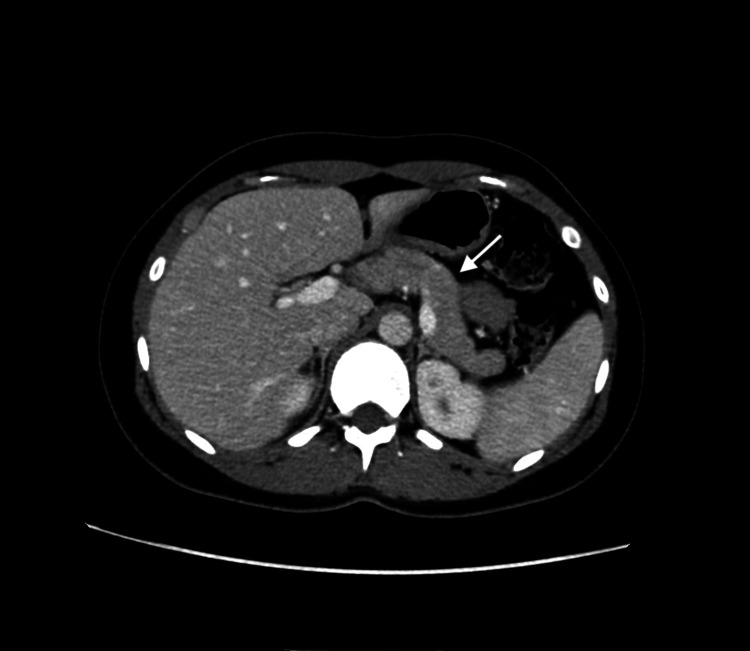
Contrast-enhanced axial CT of the abdomen displaying normal findings of the pancreas. Axial contrast-enhanced CT of the abdomen demonstrating normal pancreatic morphology without focal lesions, helping exclude insulinoma.
